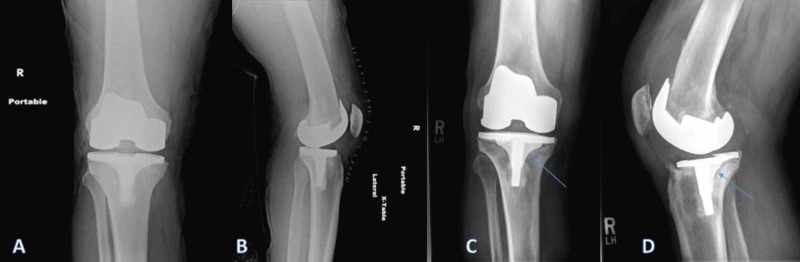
Right knee anteroposterior (AP) and lateral (LAT) radiographs taken immediately after the initial surgery (A, B) in comparison with those taken just prior to the revision surgery (C, D) showing minimal periprosthetic osteolytic areas underneath the tibial base plate with well-fixed cement-bone interface on the rest of the implant but no signs of metal-cement de-bonding
